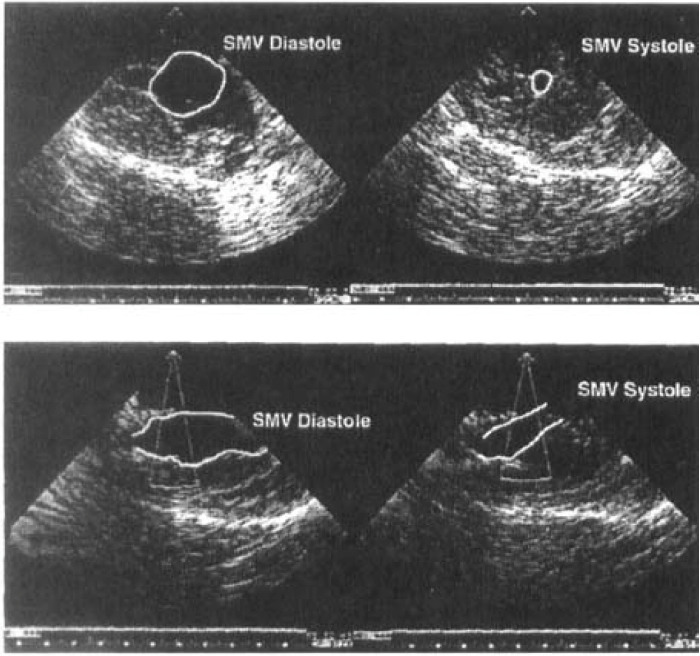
Echocardiographic examination of the skeletal muscle ventricle
